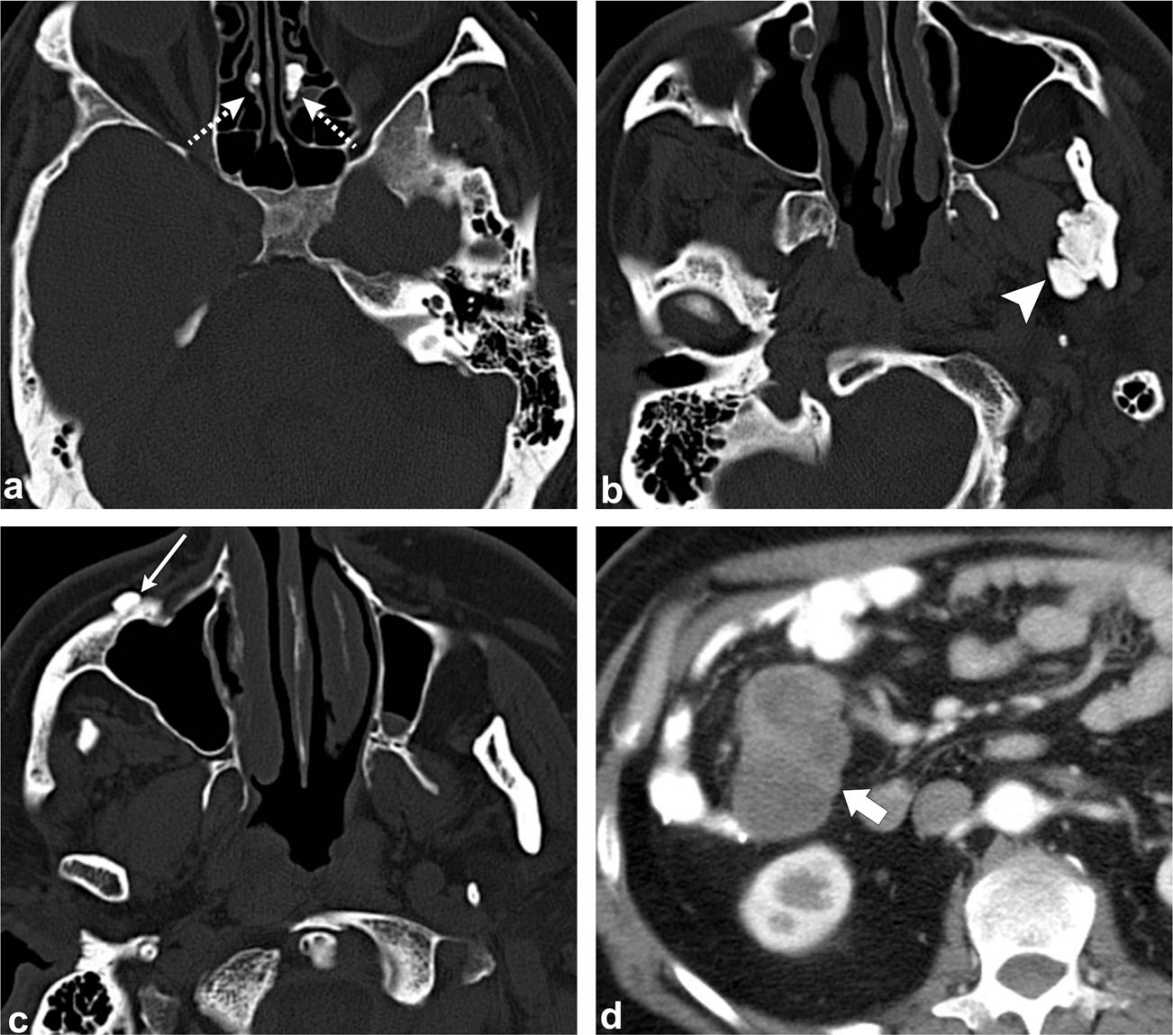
Gardner Syndrome (multiple osteomas). Axial head CT images (a–c) show multiple osteomas in the ethmoid air cells (*dashed arrows*), left ramus of the mandible (*arrowhead*) and right anterior wall of the maxillary sinus (*thin arrow*). This patient was also found to have a mesenteric desmoid tumour (*thick arrow*, d)
